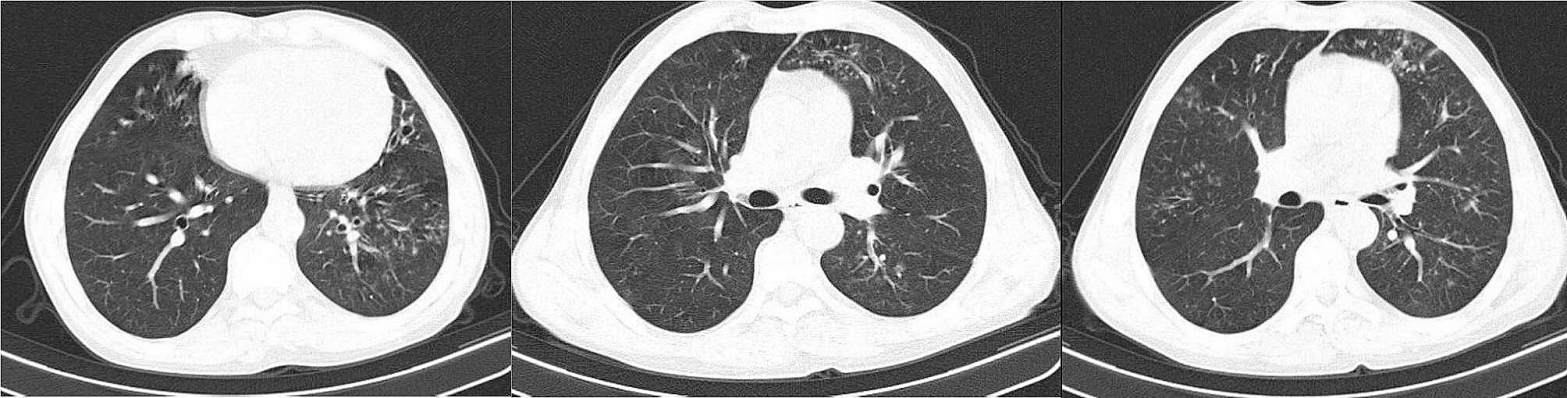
Lung CT scan of 45 years old man with lophomoniasis. Tree-in-bud in right upper lobe (RUL), right middle lobe (RML), right lower lobe (RLL), left upper lobe (LUL), lingula, right upper lobe (LLL) and bronchiectasis in lingula
